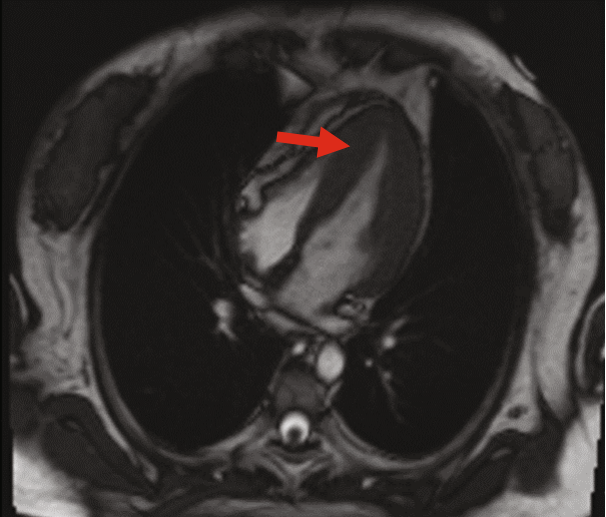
Cardiac magnetic resonance showing the walls of the left ventricle were significantly thickened, mainly in the middle and lower segments (T2-weighted image).
